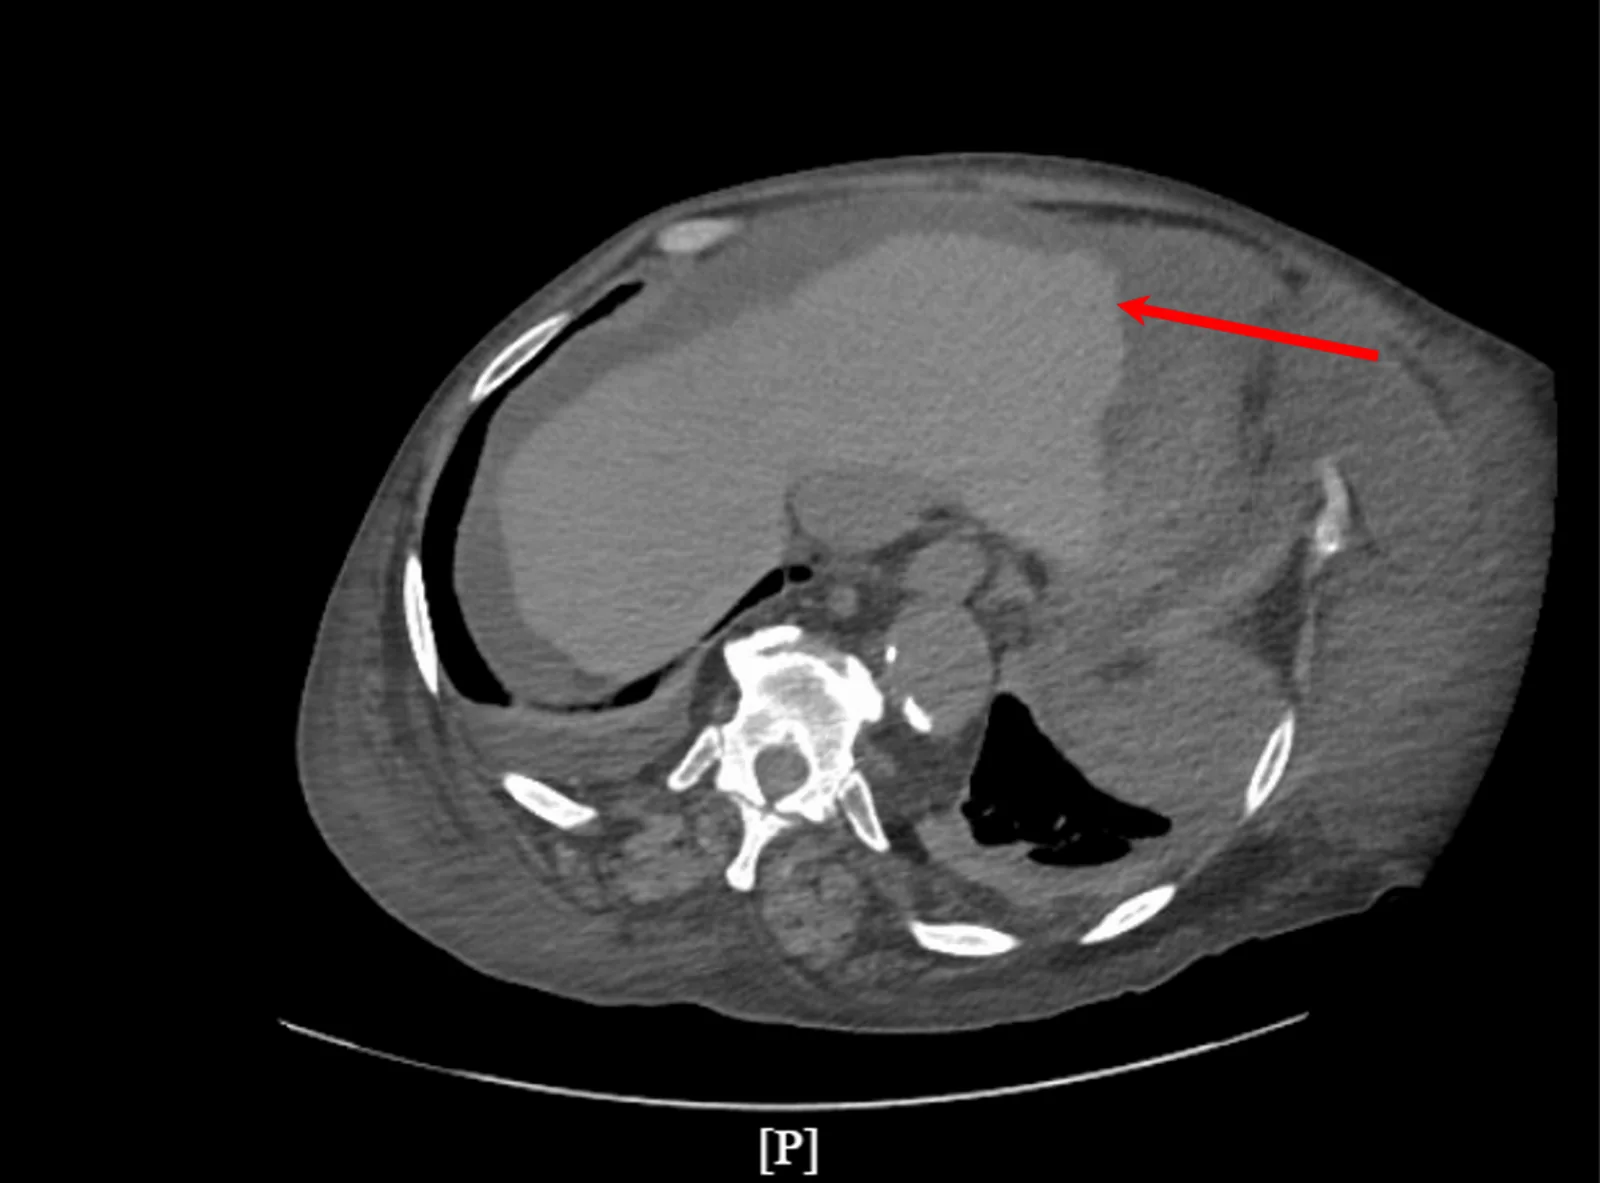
CT of the abdomen demonstrating cirrhotic liver morphology Axial CT image showing a nodular hepatic contour consistent with cirrhotic morphology (arrow) with associated abdominal ascites. CT: computed tomography
